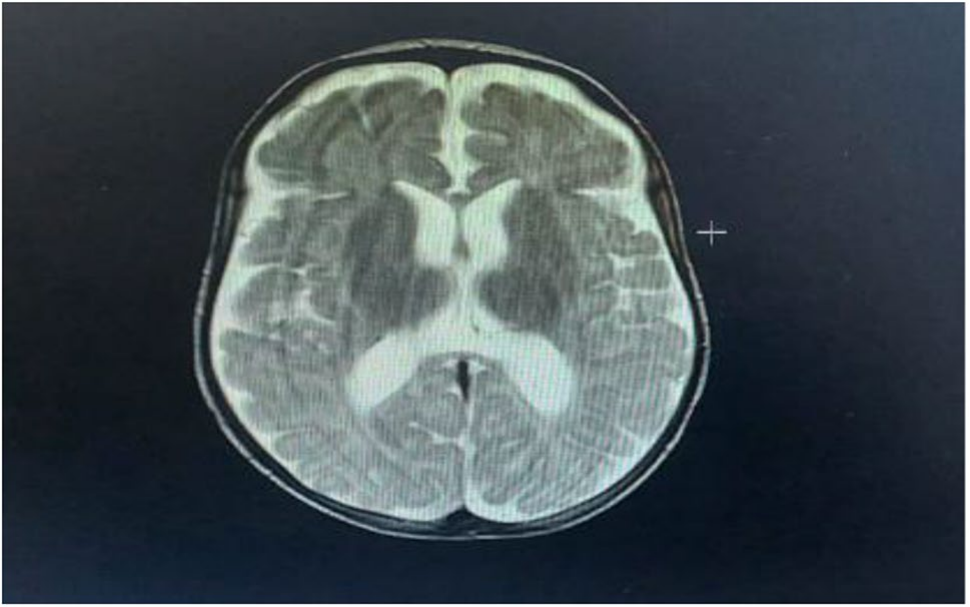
MRI brain of the patient (Note prominent cortical sulci and scant deep periventricular white matter)
